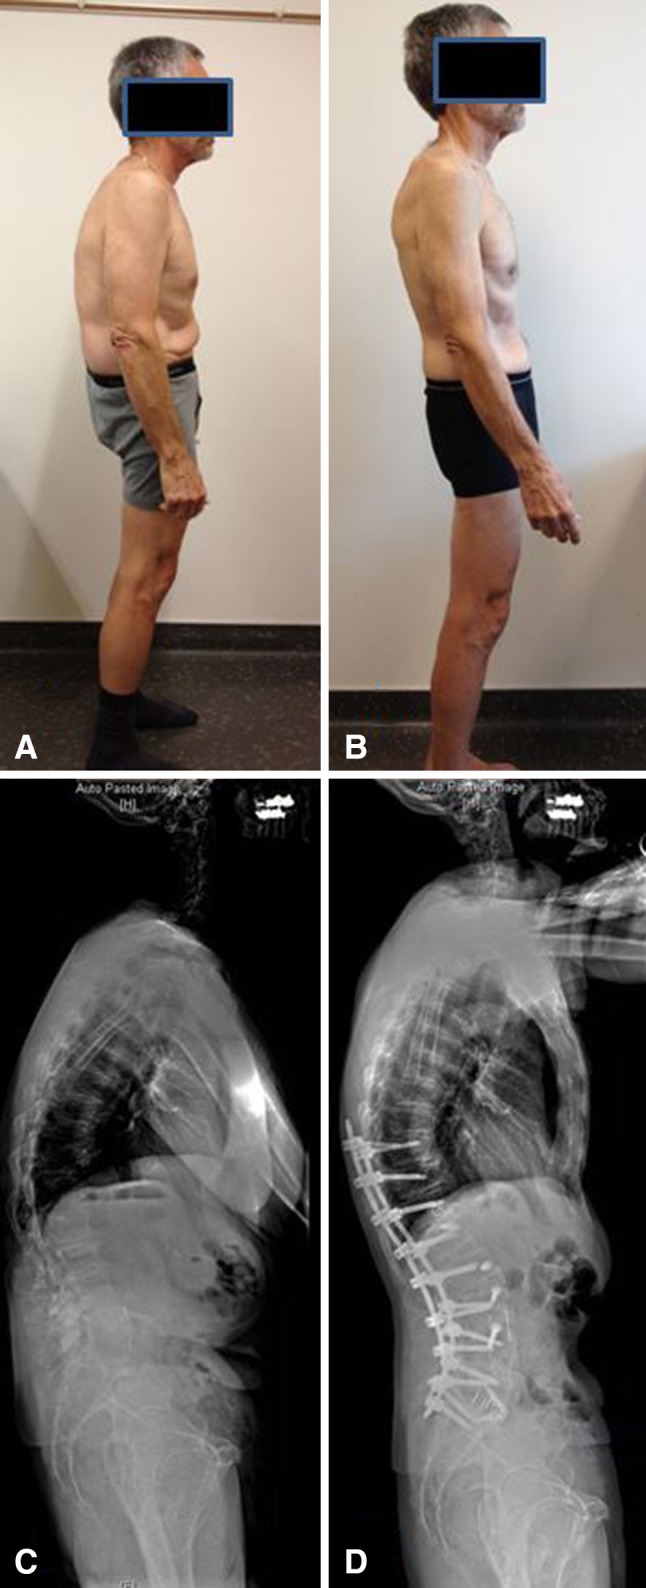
The patient is a 67-year-old man who initially presented to the clinic with severe back and leg pain and limited walking ability. Preoperative sagittal balance, ODI, and VAS back/leg pain were 116 mm, 50%, and 4.7, respectively. The patient was treated with the T10-L5 MIS lateral approach, L5-S1 ALIF, T12L1, L23, L34 anterior longitudinal ligament release with a hyperlordotic cage placed at those levels, and T10-S1 percutaneous pedicle screws. Two-year followup sagittal balance, ODI, and VAS were 34 mm, 2%, and 2, respectively. (A) Preoperative side-view photograph. (B) Postoperative side-view photograph. (C) Preoperative lateral radiograph. (D) Two-year followup lateral radiograph.
